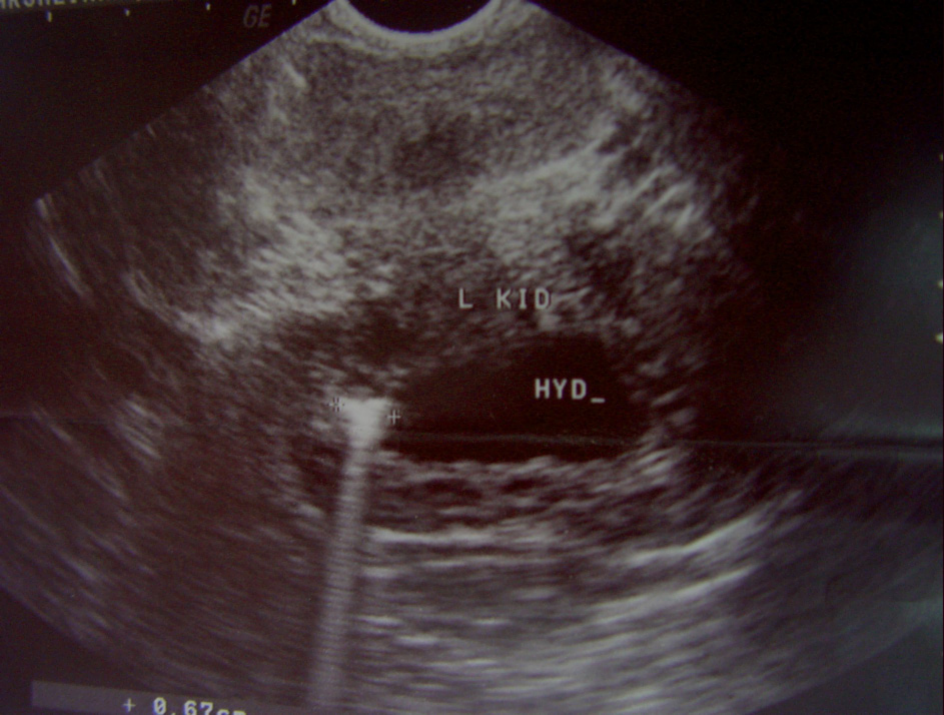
Transrectal ultrasonogram of a left side pelvic mass behind the bladder. Note the thick wall of the cystic shaped mass and the echogenic particle inside with the posterior shadow.
